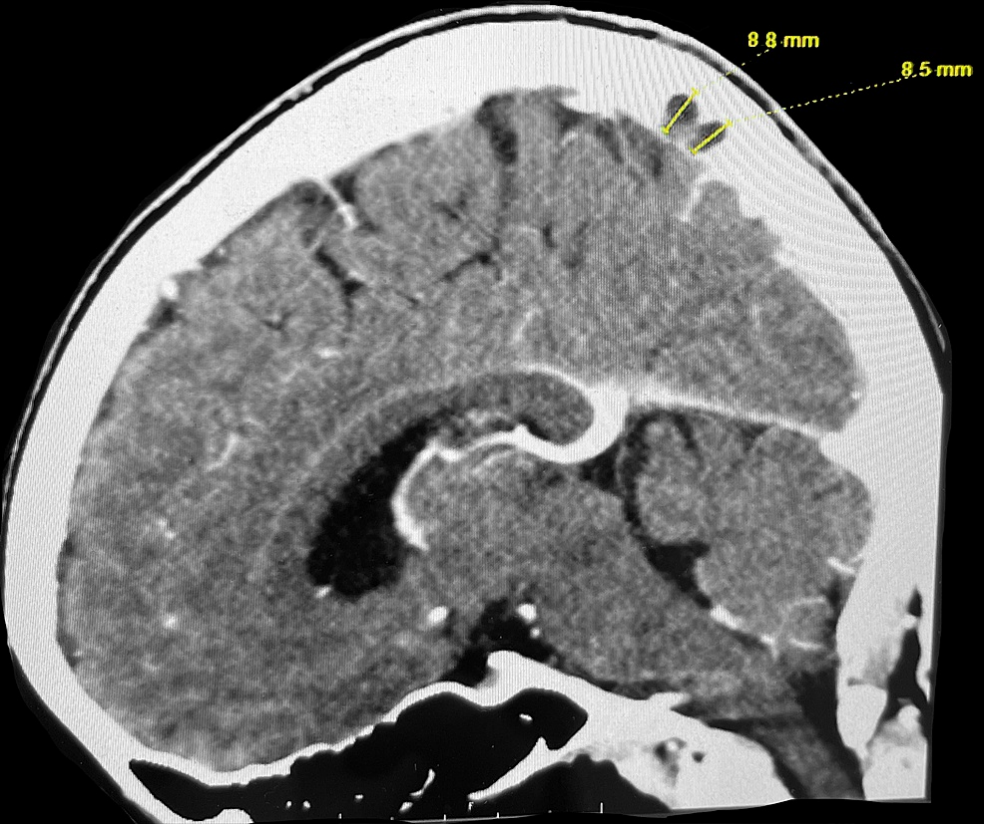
CT Venogram With Sagittal Sinus Filling Defects
